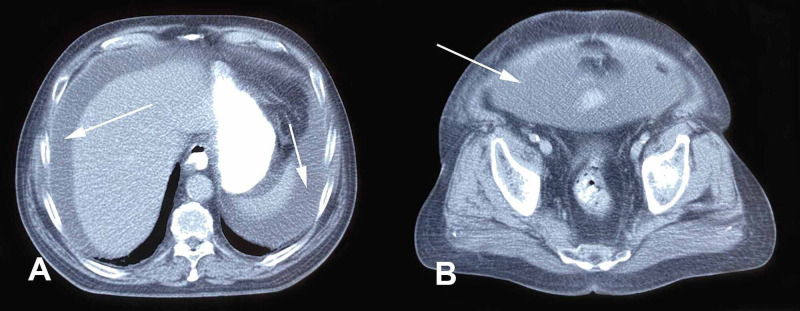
CT of the upper (A) and lower (B) abdomen showing the presence of free fluid (arrows). CT, computerized tomography
